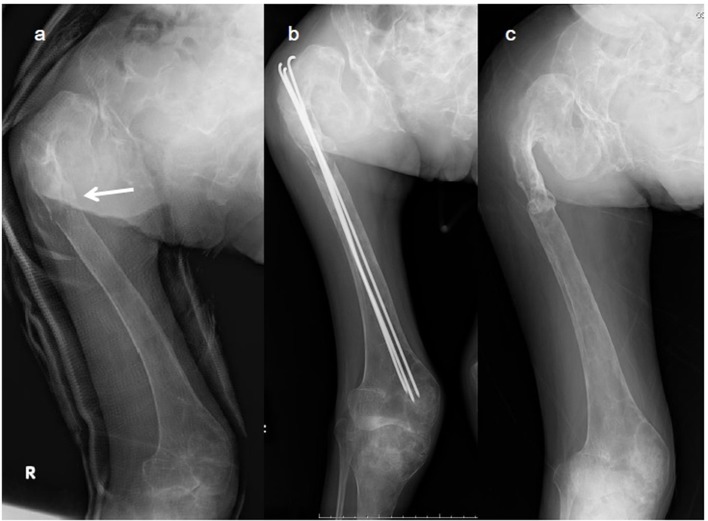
X-ray images of the right femur. (a) Preoperative radiograph of the right femur showing a pathological subtrochanteric femoral fracture (arrow) and a diffuse fibrodysplastic lesion of the entire femur with the typical shepherd’s crook deformity. (b) Femoral fracture was fixed percutaneously with three titanium Kirschner wires through the great trochanter to the distal femoral condyle. (c) Radiograph 1 year after the procedure confirming bony union.
